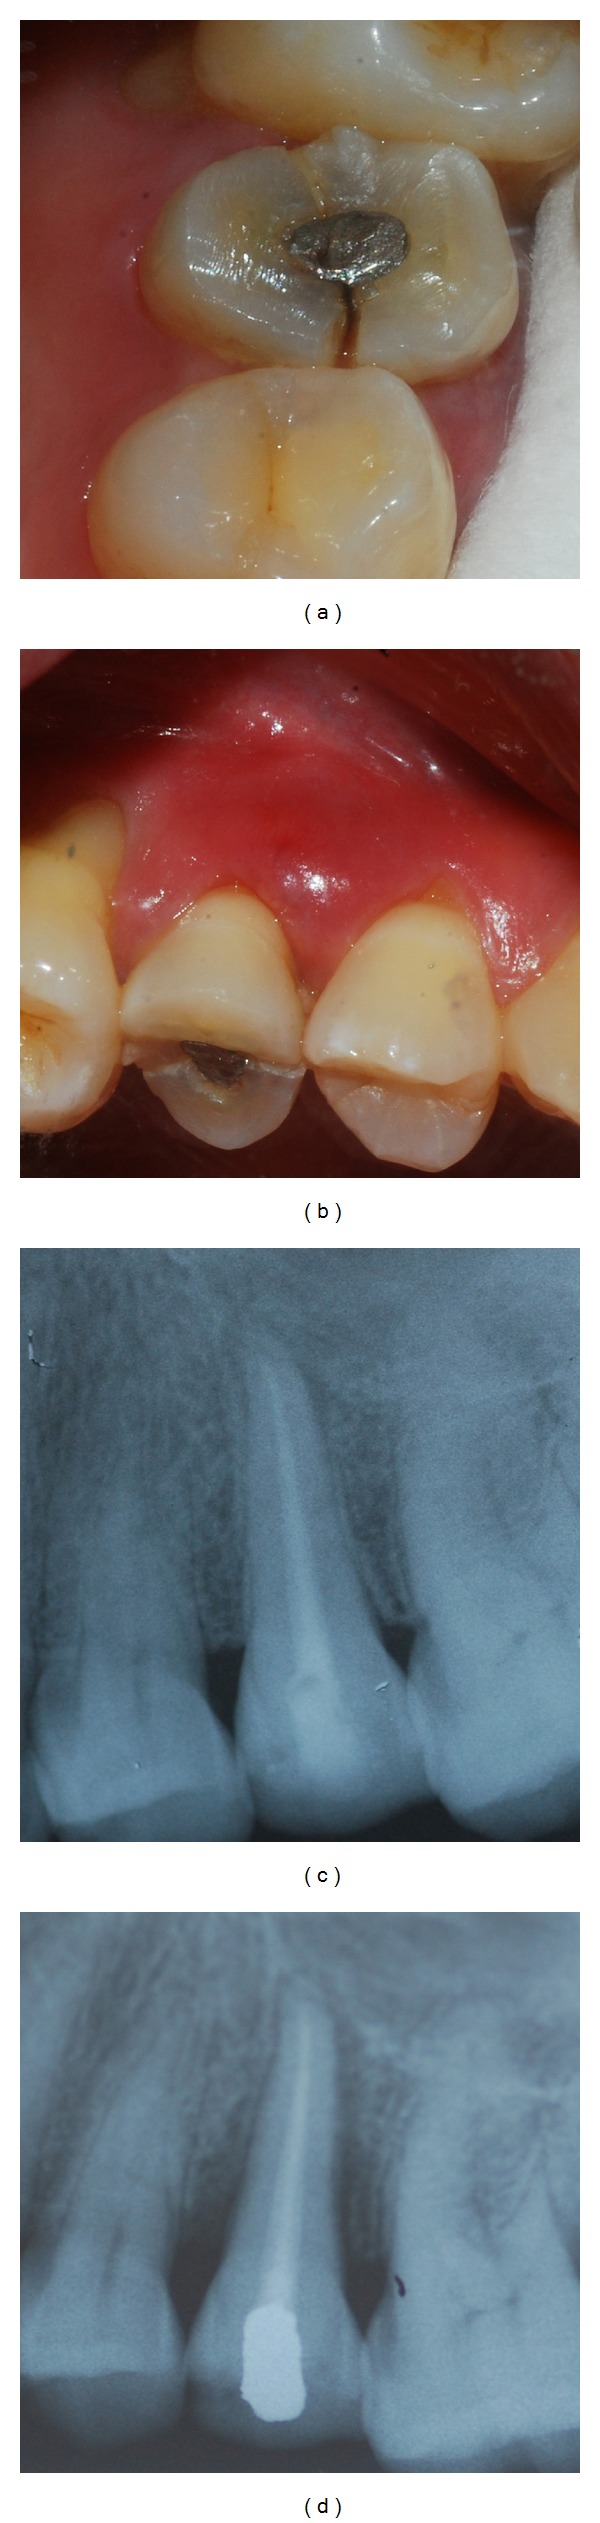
(a) Fracture extending mesiodistally on centre of occlusal surface and then onto proximal surface of 25. (b) Diffuse swelling of the buccal gingiva. (c) Post obturation intraoral periapical radiograph of 25. (d) Intraoral periapical radiograph taken after 15 months shows severe angular bone loss interproximally associated with propagation of fracture line.
